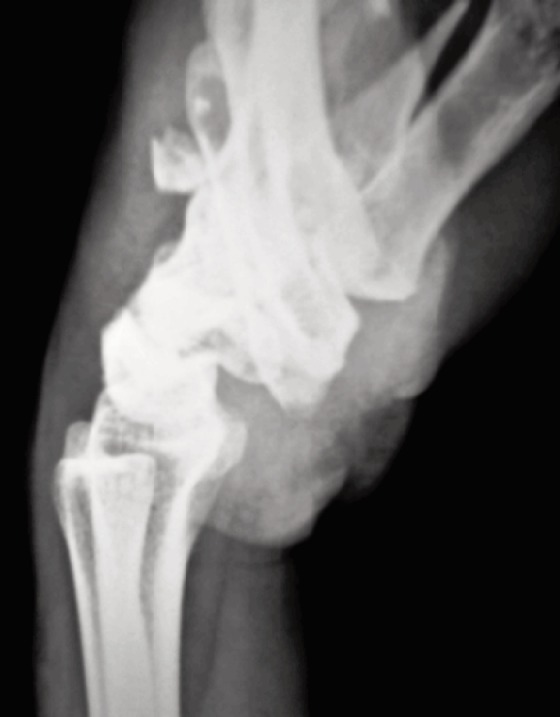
Lateral radiograph view showing Classification Type B.1. Volar dislocation of multiple metacarpals along with concomitant fracture at the base of ring metacarpal shaft.
